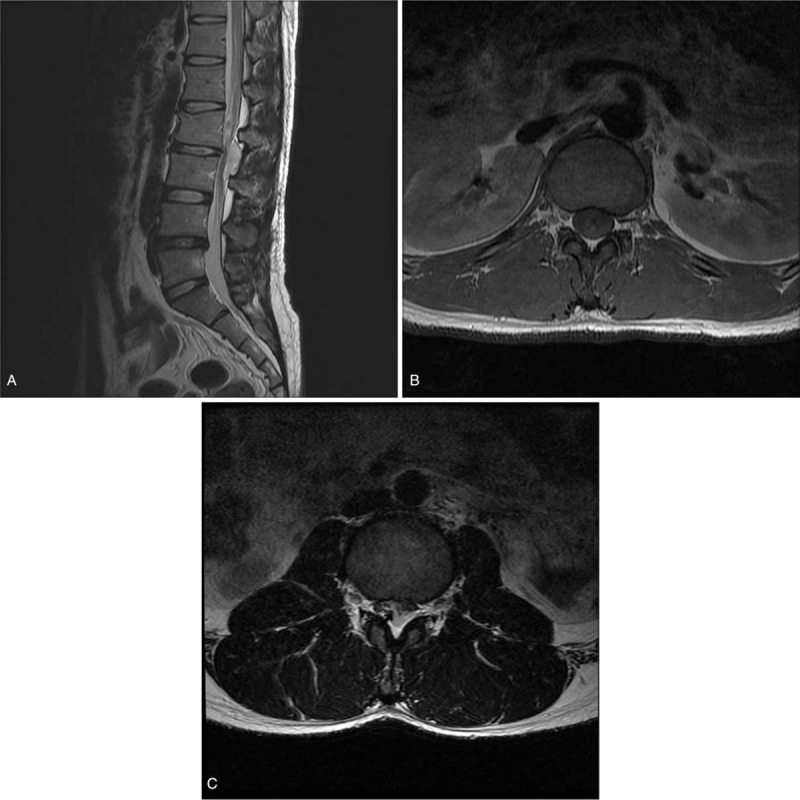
Sagittal T2-weighted (A) MRI demonstrating a compression fracture of the L2 body at the upper anterior portion and a mass-like lesion on the intradural and posterior epidural area at L2-L3 level. Axial T1-weighted (B) and T2-weighted (C) MRI showed a deviation of the cauda equina by an intradural mass-like lesion and a lesion affecting the nerve roots and thecal sac. Two lesions (an intradural mass-like lesion on the ventral side of the spinal cord and an epidural mass-like lesion on the dorsal side) were suspected of connectivity (arrow). MRI = magnetic resonance imaging.
